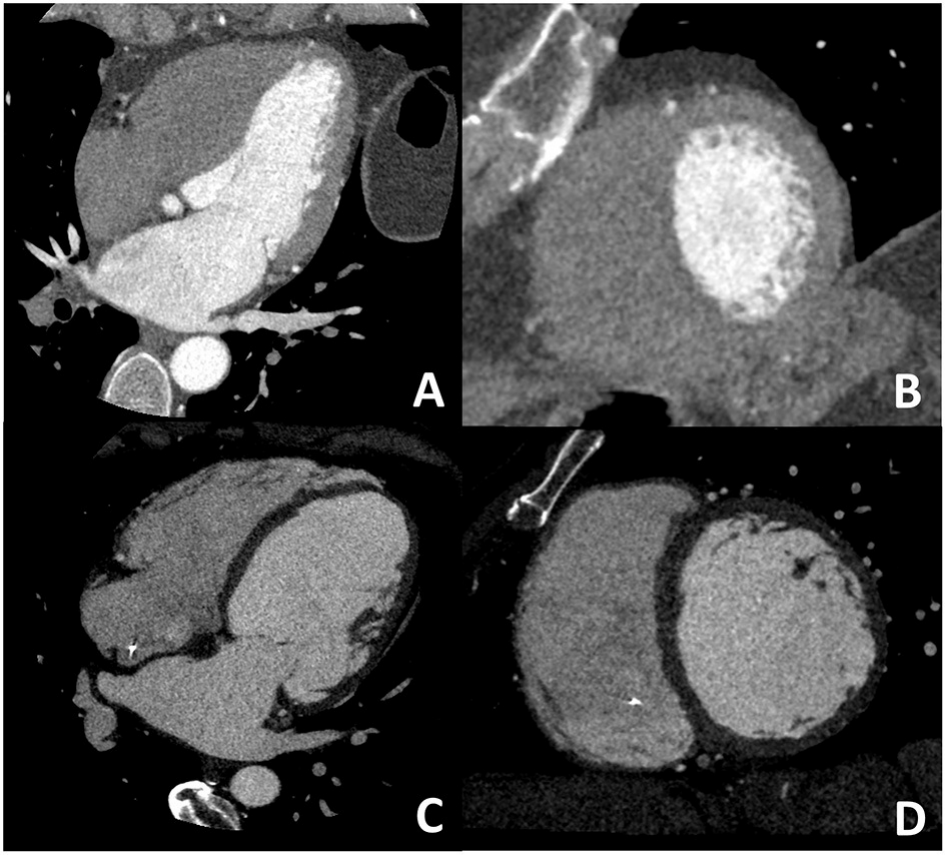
Dedicated cardiac CT (CCT) scan protocol for the comprehensive evaluation in DCM patients. In (A,B) a cardiac CT dedicated to coronary anatomy evaluation is shown; it is well evident how it is not possible to correctly identify the right ventricular border of the interventricular septum. In (C,D) a CT with dedicated acquisition protocol for biventricular volume and function in which all four chambers are opacified by contrast medium enabling correct right ventricular border identification. These examples highlight the need for a dedicated acquisition protocol for most of the non-coronary cardiac CT findings.
